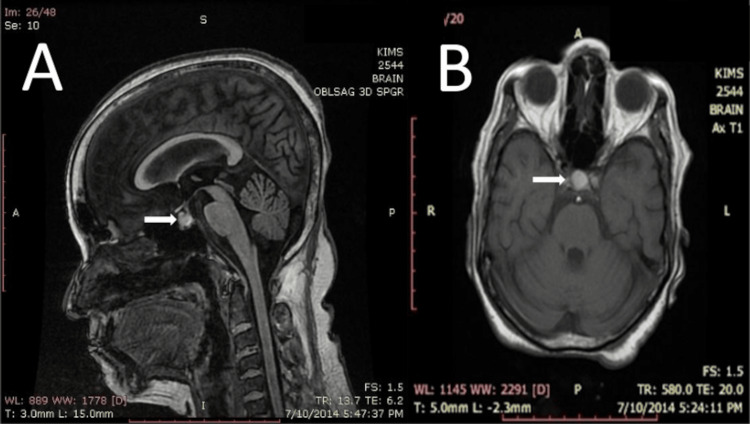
Case 2: (A) Sagittal T1-weighted (SPGR sequence) MRI shows a heterogeneously hyperintense anterior pituitary mass (arrow). (B) Corresponding axial T1-weighted MRI (SPGR sequence) image of the same patient shows a discrete rounded hyperintense mass (arrow) suggestive of haemorrhagic pituitary macroadenoma of size 0.7 cm x 0.9 cm x 1.1 cm (anteroposterior x transverse x craniocaudal). Abbreviations: SPGR, spoiled gradient recalled
